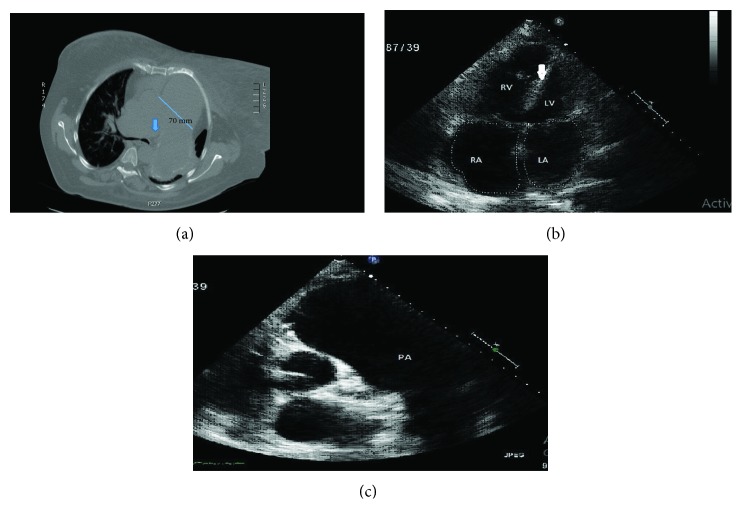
(a) Noncontrast chest CT showing main pulmonary artery aneurysm measuring 70 mm with blue arrow pointing the possible intimal flap. Rapid tapering of pulmonary vessels can be appreciated on the right lung zones. (b) Transthoracic echocardiogram apical four-chamber view showing severely dilated right ventricle and septal flattening (white arrow). (c) Transthoracic echocardiogram parasternal short axis view at the level of aortic valve showing severe dilation of pulmonary artery.
